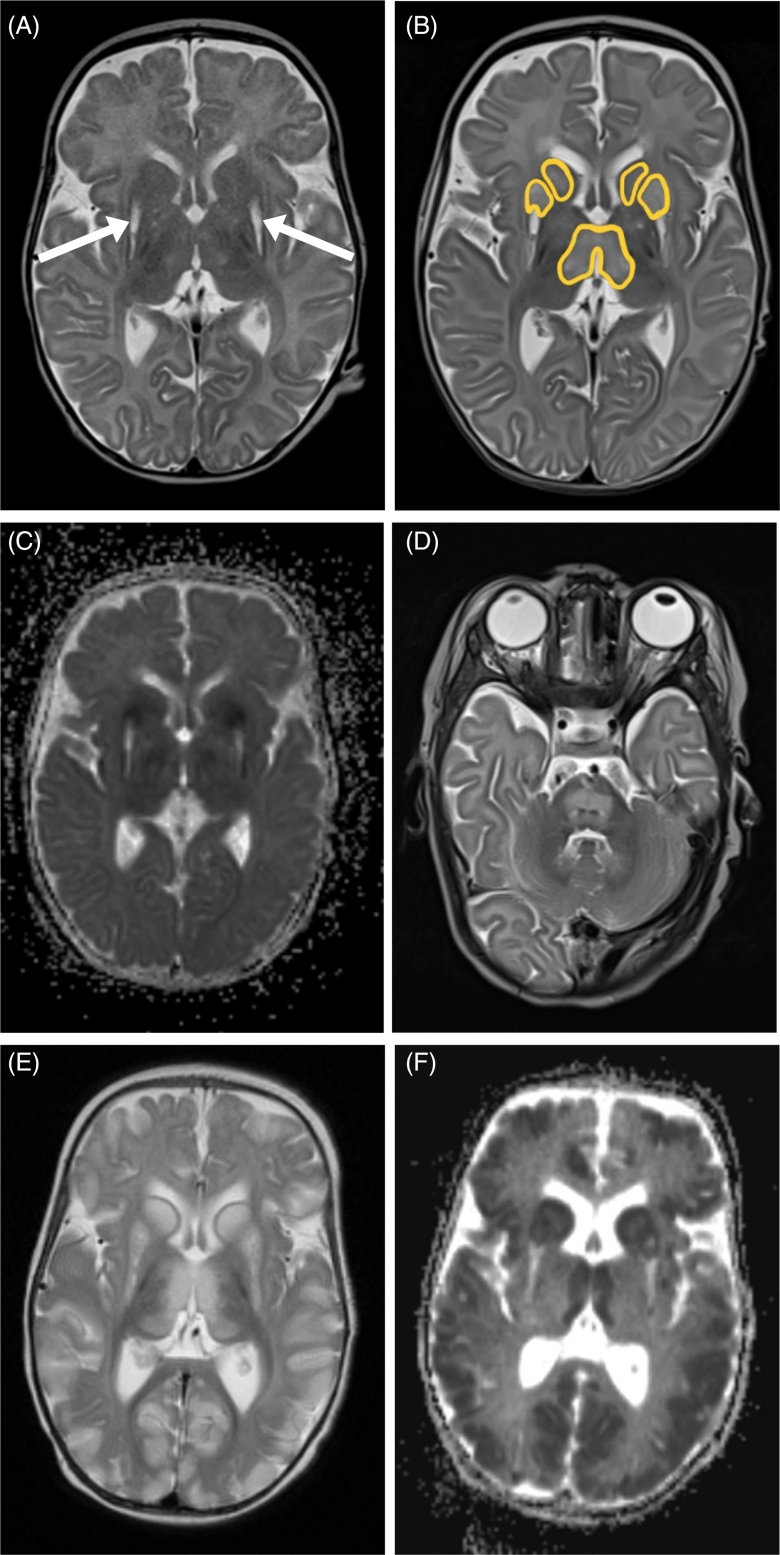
Cranial MRI at three different points of time: A, B through D, E, and F. (A, B, D, E) T2‐weighted images; A ‐ The white arrows indicate the symmetric lesions in both putamina, typically found in individuals with PA; B ‐ New hyperintense lesions in basal ganglia marked in yellow; D ‐ Symmetric pontine hyperintensity; E ‐ New massive, hyperintense lesions involving cerebral cortex. (C, F) Diffusion‐weighted imaging; black areas indicating cytotoxic edema.
